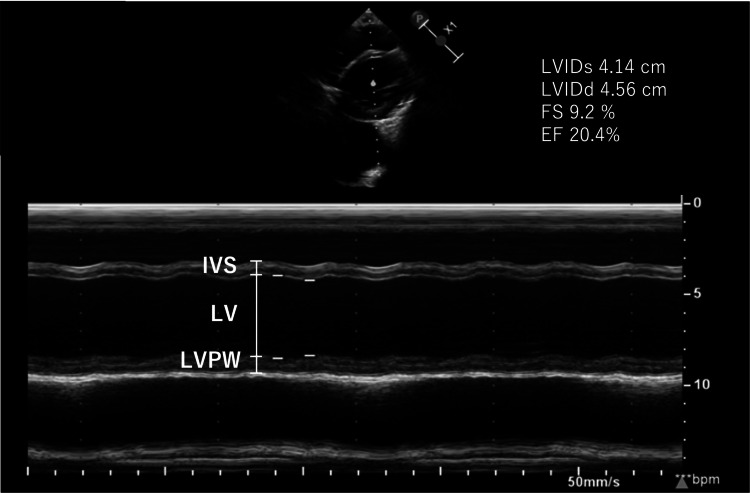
TTE M-mode image at admission. Transthoracic echocardiography was performed using a PHILIPS EPIQ5G ultrasound system (Amsterdam, The Netherlands: Philips) equipped with an S8-3 broadband sector array transducer (3.0-8.0 MHz). TTE: transthoracic echocardiography; LV: left ventricle; IVS: interventricular septum; LVPW: left ventricular posterior wall; LVIDs: left ventricular internal dimension at end-systole; LVIDd: left ventricular internal dimension at end-diastole; FS: fractional shortening; LVEF: left ventricular ejection fraction
